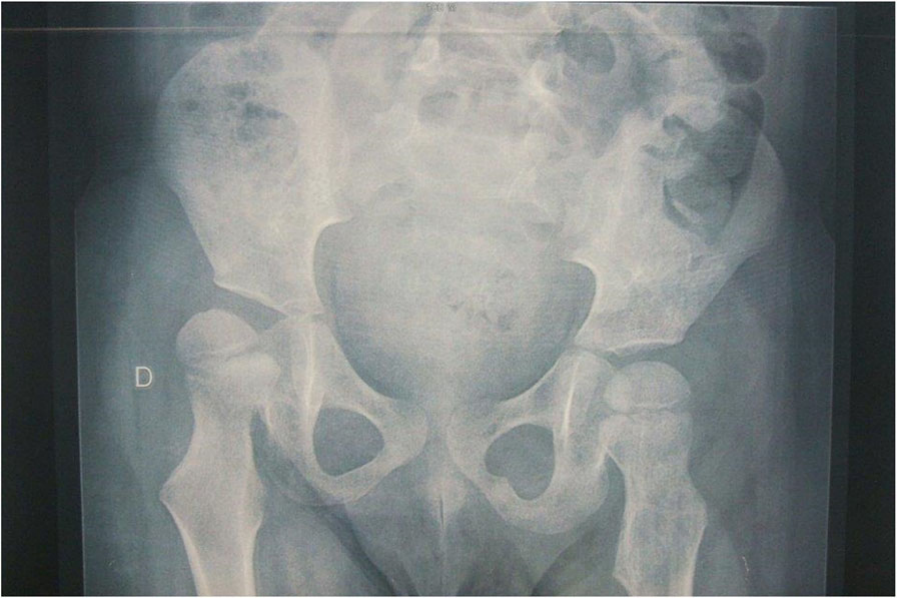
AP pelvis radiograph demonstrating classic signs of neuromuscular hip dysplasia including coxa valga, caput valgum, acetabular dysplasia, and subluxation of the hip joint
